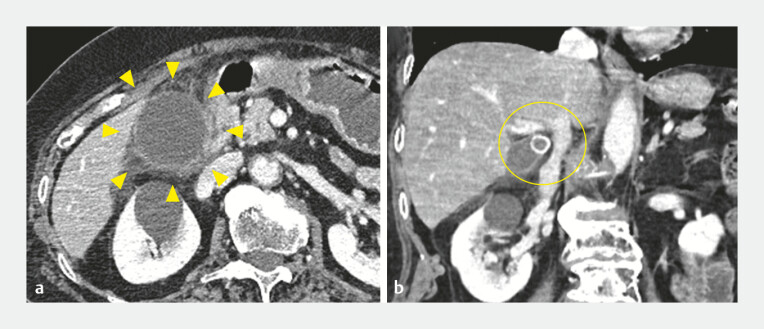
Contrast-enhanced computed tomography at the time of diagnosis of acute cholecystitis.
